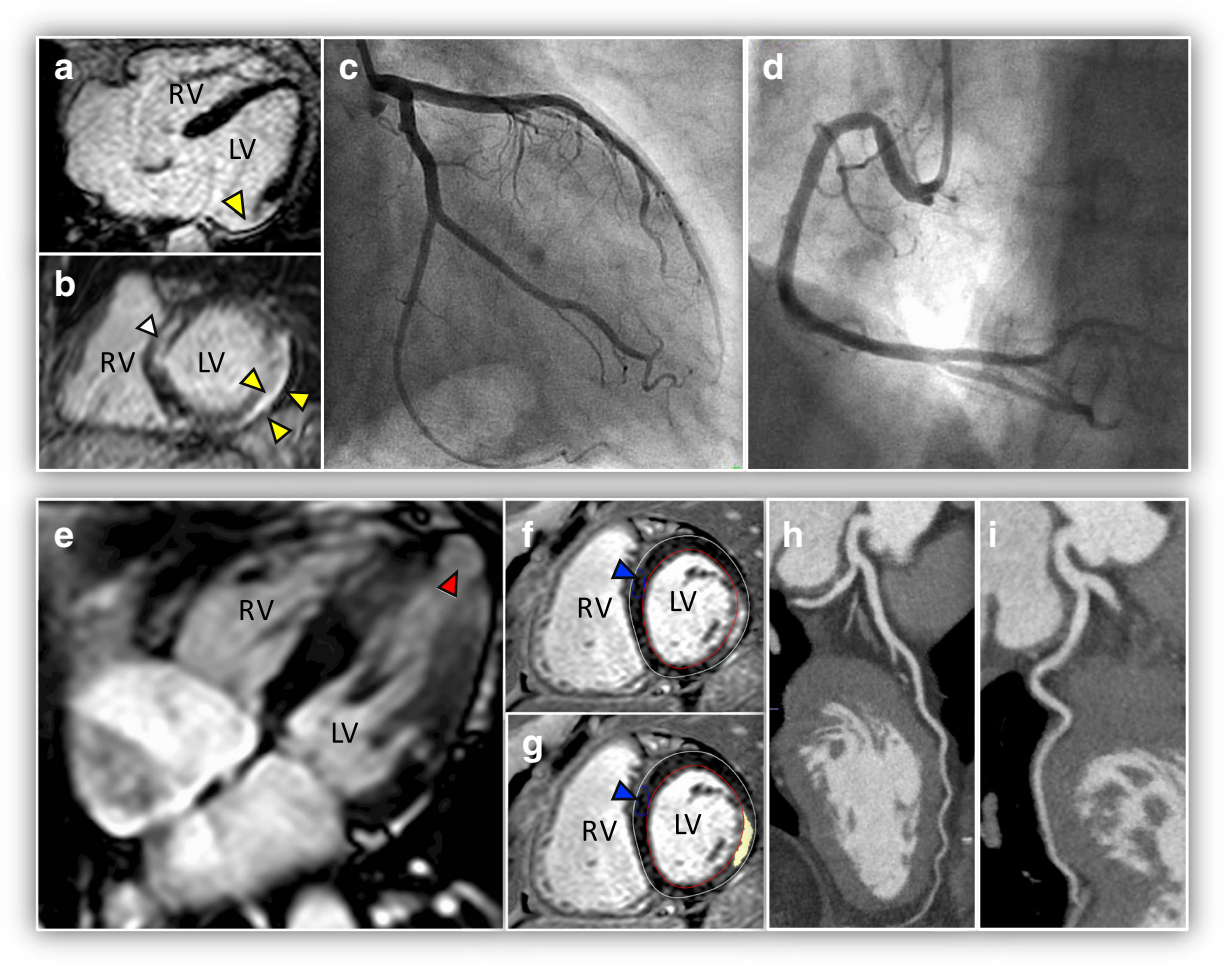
Examples of CMR, invasive and CT angiography in two representative patients with Chagas’ heart disease. *First patient (top row)*: a and b CMR reveals transmural LGE pattern in the LV lateral wall (*yellow arrow*) with concomitant septal midwall LGE (*white arrow*) and, by invasive angiography, (c and d) normal coronary arteries. *Second patient (bottom row):* (e) cine-CMR image reveals a classical Chagas’ heart disease finding, the vorticle aneurysm (*red arrow*), (f and g) LGE reveals subendocardial pattern in the lateral wall (*yellow arrow*) and, by CT angiography, (h and i) normal coronary arteries. Blue arrow (f and g) indicating the region of interest (ROI) for calculating the mean signal intensity (SI) of the reference normal myocardium
